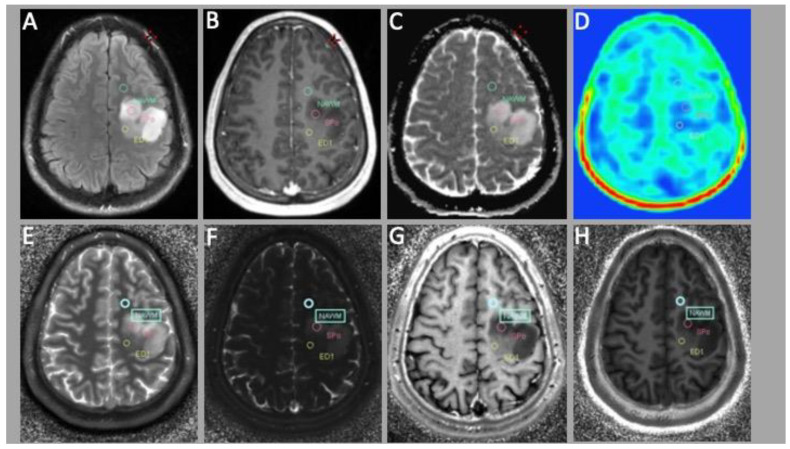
Patient with an astrocytoma Gr. 2 according to the WHO 2021 classification with a MET PET-negative tumor. From upper left to lower right: conventional MRI sequences—(A) FLAIR (Fluid attenuated inverse recovery); (B) T1w images with contrast enhancement; (C) ADC map; and (D) MET PET image. MRF images—(E) T1 map; (F) T2 map; (G) R2 map; and (H) R1 map.
